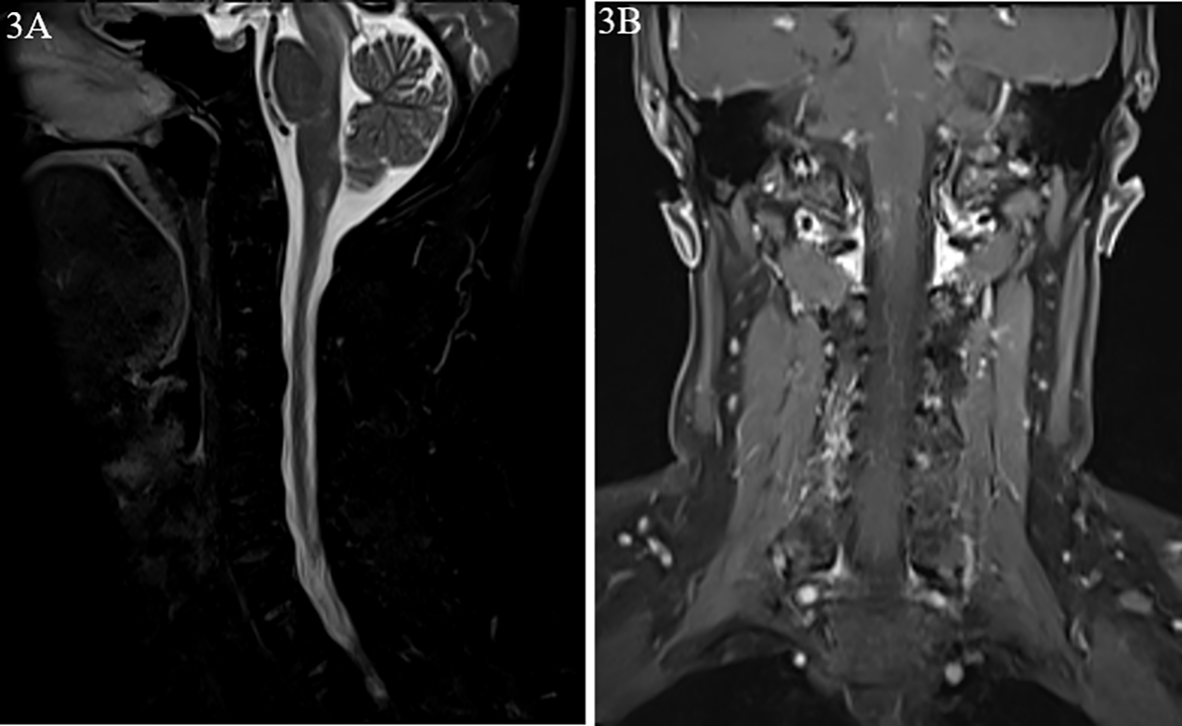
Magnetic resonance images from case 2. (A) Hypersignal of the brain stem and the area postrema on T2-weighted image. (B) Nodular enhancement on dynamic enhanced magnetic resonance imaging (MRI).
